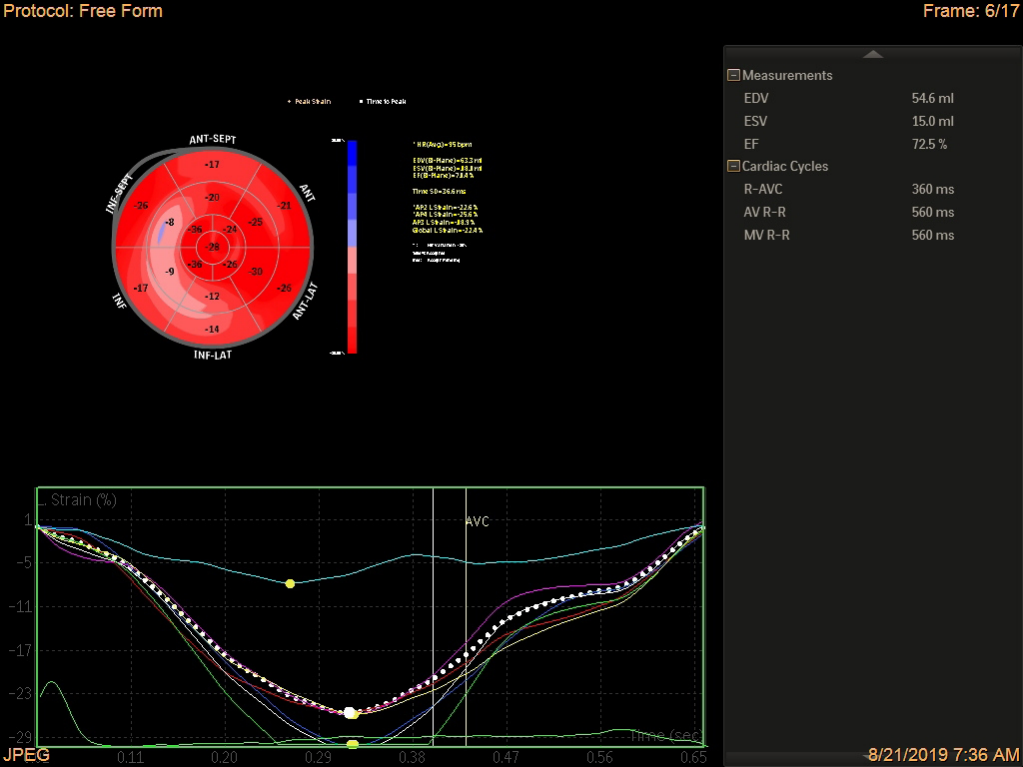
All measurement was done by single expert echocardiographist. To reduce interobserver variability, measurements were repeated in random order by an interval of 1 month. To test interobserver variability, a second skilled echocardiographist analyzed data without knowledge of the measurement of first observer.
